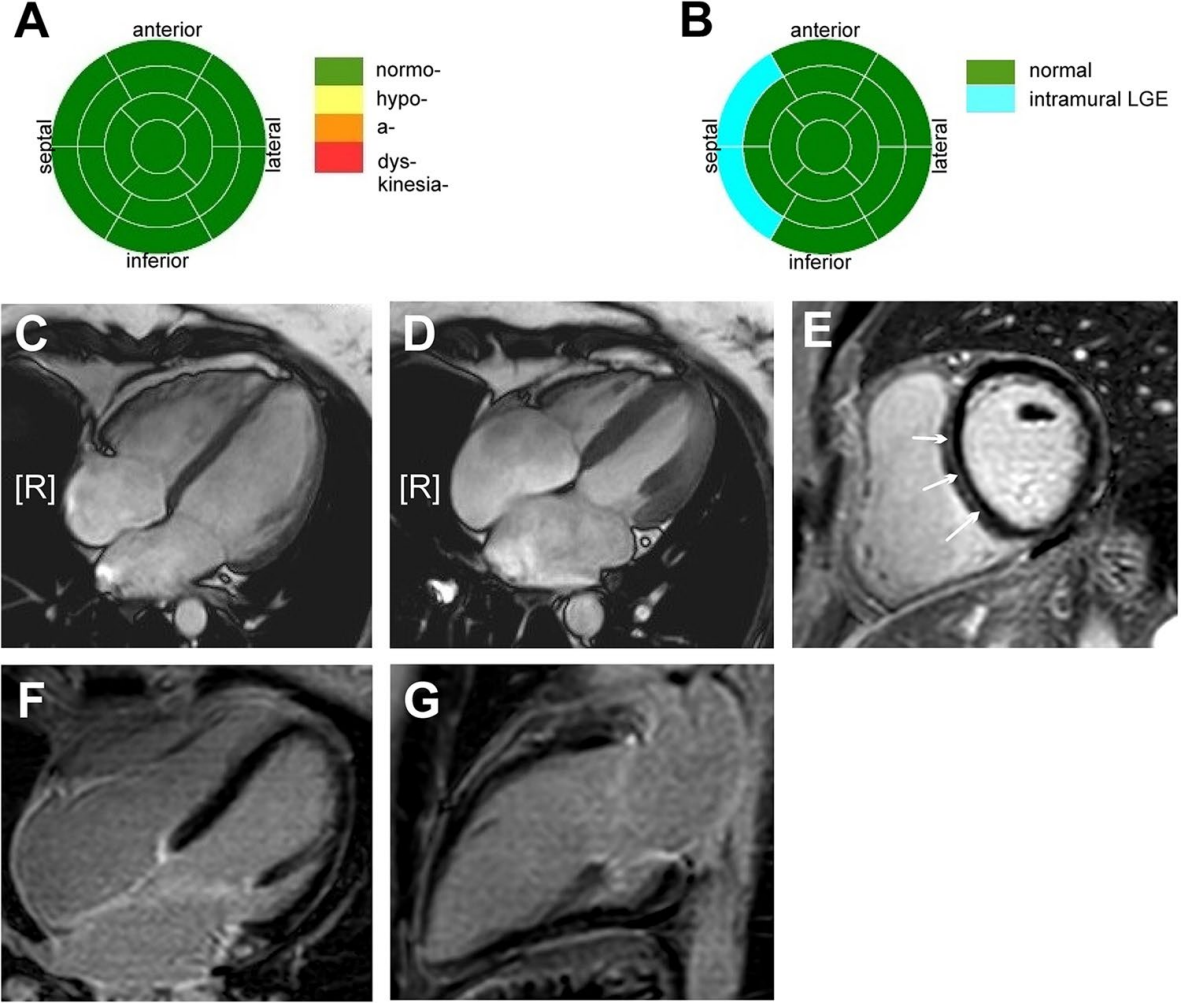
a–e The cardiac MRI prior to 2-lead ICD implantation: a bulls eye blot with normal wall movements with a normal left ventricular ejection, b bulls eye blot of typical LGE pattern in the intramural septum, c enddiastolic and d endsystolic four chamber view and e short axis view of typical midwall fibrosis with LGE (marked with white arrows). f LGE in four chamber view, g LGE in two chamber view: Also in the atria (LA > RA) an LGE signal typical for atrial fibrosis can be detected
